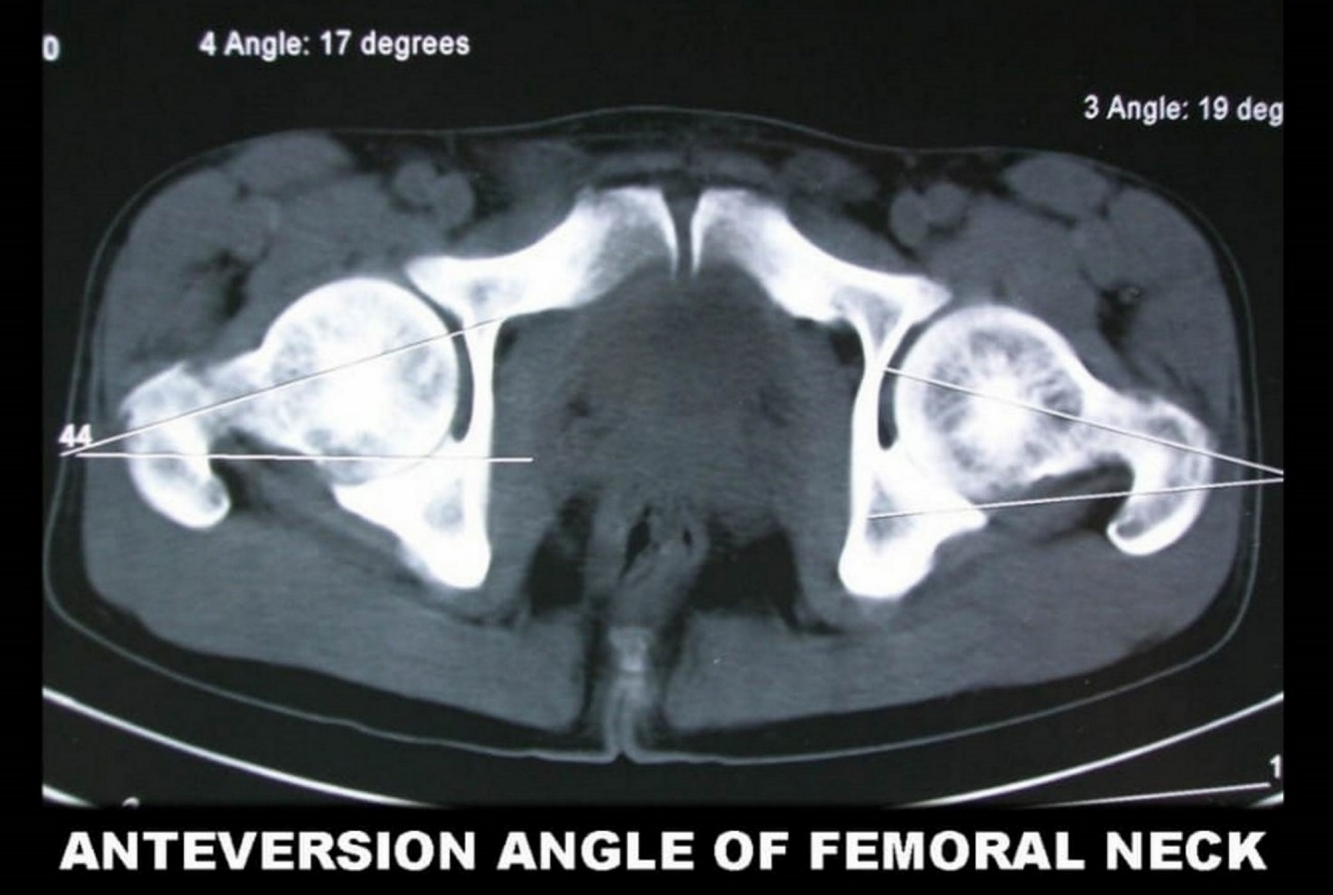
Anteversion angle of femoral axis calculated from computed tomography
